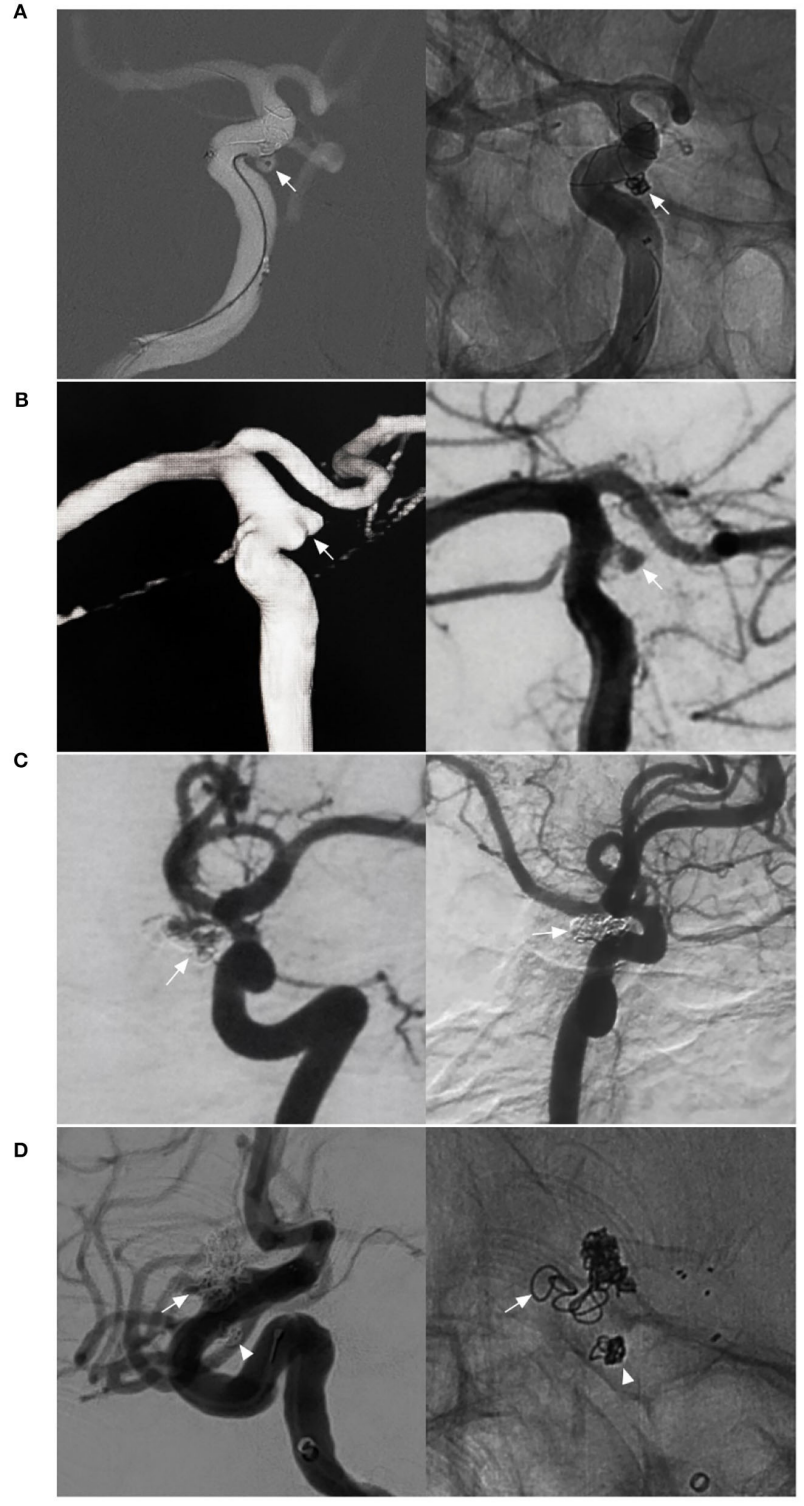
EVT difficulty in aneurysms of the paraophthalmic segment. (A) Left, Road map navigation shows the microcatheter tip (arrow) positioned in the aneurysm sac with the assistance of stent semi-deployment. The stent sealed a part of the aneurysm neck and provided the opportunity for the microcrater to enter the aneurysm; right: unsubtracted DSA shows the coils in the aneurysm (arrow). (B) Left, three-dimensional DSA shows a wide-necked lobulated carotid cave aneurysm (arrow); right: DSA shows the loose packing of the coiling in the aneurysm because the microcatheter was knocked off the aneurysm, but contrast agent retention can be seen in the aneurysm (arrow). (C) Left, DSA shows a recanalized aneurysm due to low-density packing (arrow); right: follow-up DSA shows the aneurysm had a complete embolization (arrow) after repeated coiling. (D) Left, DSA shows a recanalized lobulated upper aneurysm (arrow) and a completely embolized lower aneurysm (arrowhead); right: X-ray film shows that the upper aneurysm had low-density packing due to difficult catheterization (arrow), and the lower aneurysm had satisfactory embolization (arrowhead). DSA, Digital subtraction angiography; EVT, endovascular treatment.
